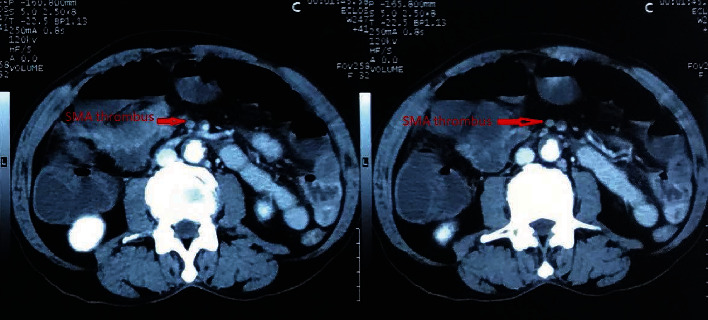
CT scan depicting SMA thrombosis.
